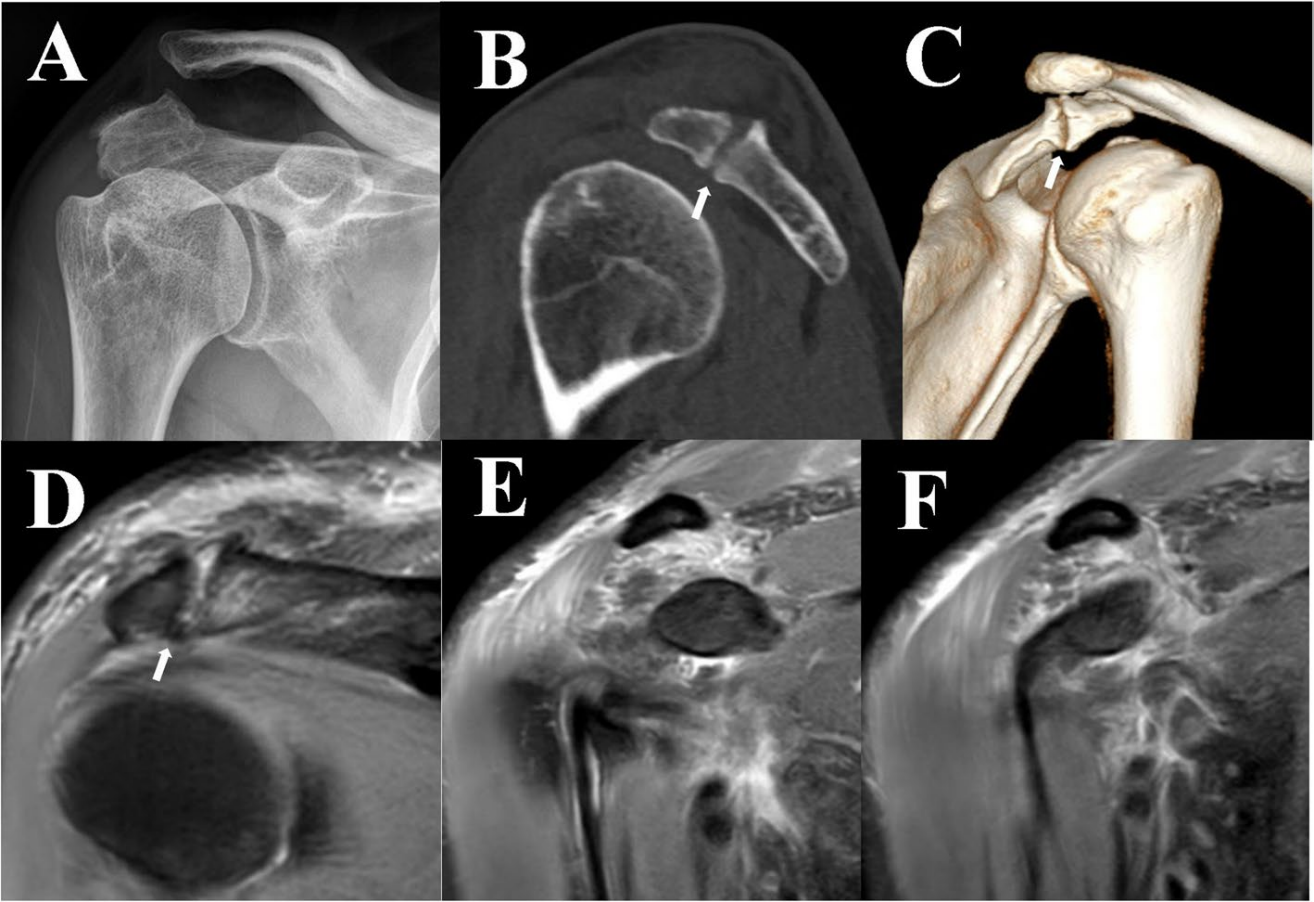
Preoperative examination of the right shoulder. A Right shoulder AP view: The increase of AC distance. B, C CT of the right shoulder: clear sclerotic margin between the os acromiale and the truncated acromion. D Pre-operation MRI: the interface between the os acromiale and the truncated acromion is likely a type of fibrocartilage. E Pre-operation MRI: discontinuity of the trapezoid ligament. F Pre-operation MRI: swollen and increased signal of conoid ligament. The white arrowhead indicates the os acromiale
